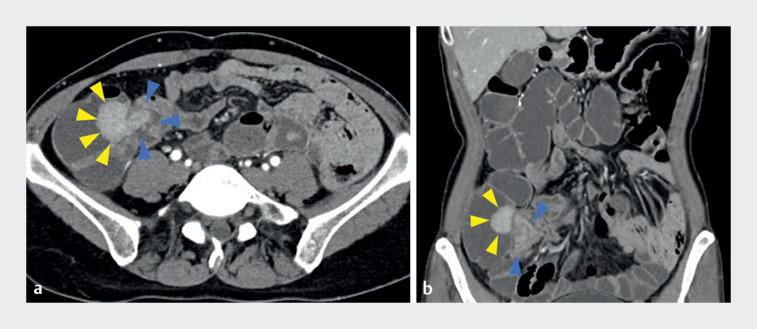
Computed tomography (CT) images of intussusception of the terminal ileum into the cecum (blue arrowheads) related to the sizable polyp (yellow arrowheads).
